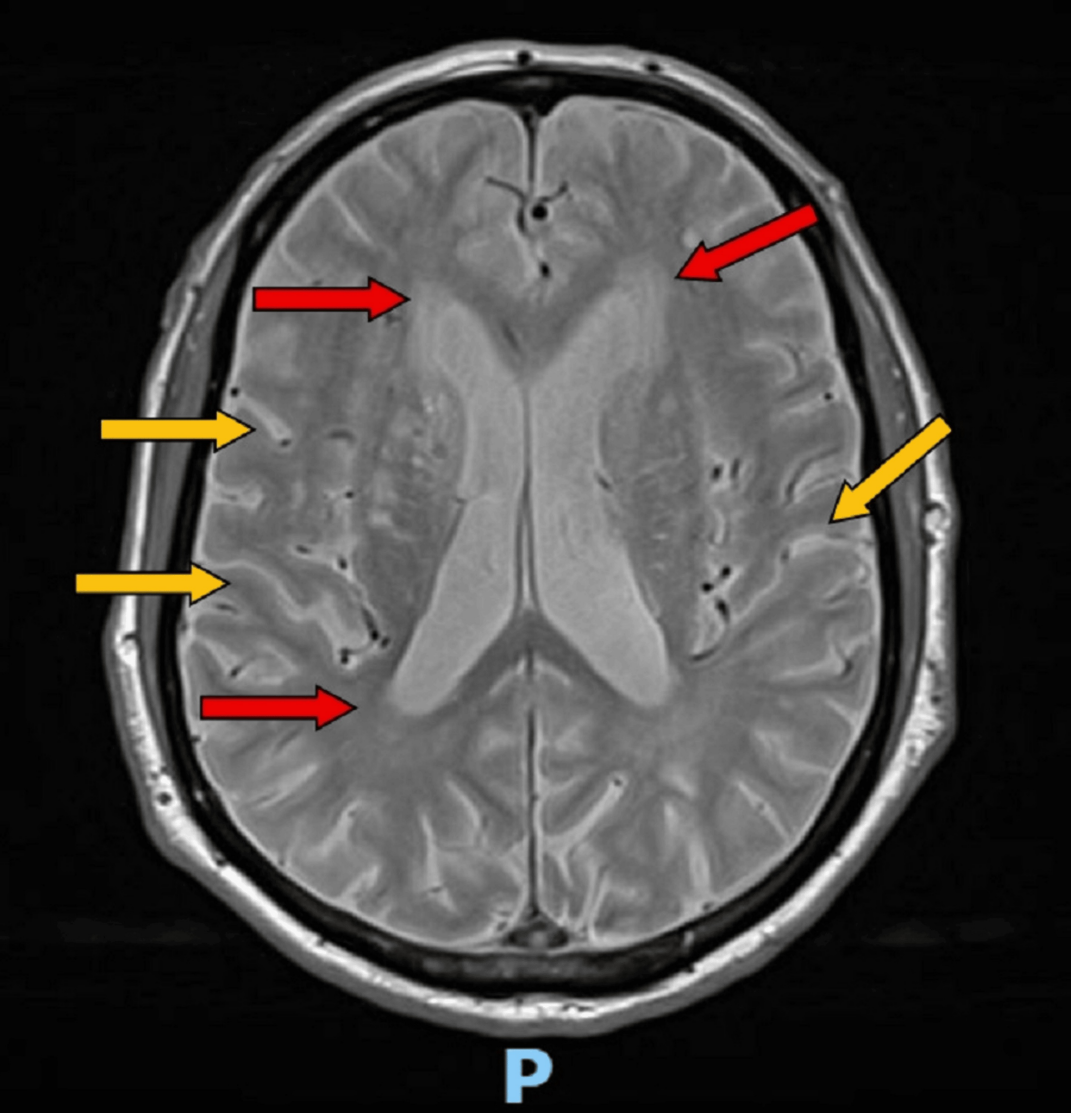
Brain MRI T2 shows no acute or space-occupying lesions. Red arrows: chronic microangiopathic white-matter disease with multiple small hemosiderin foci (likely hypertensive in origin); Yellow arrows : mild cortico-subcortical atrophy, more pronounced in the temporal and parietal regions.
